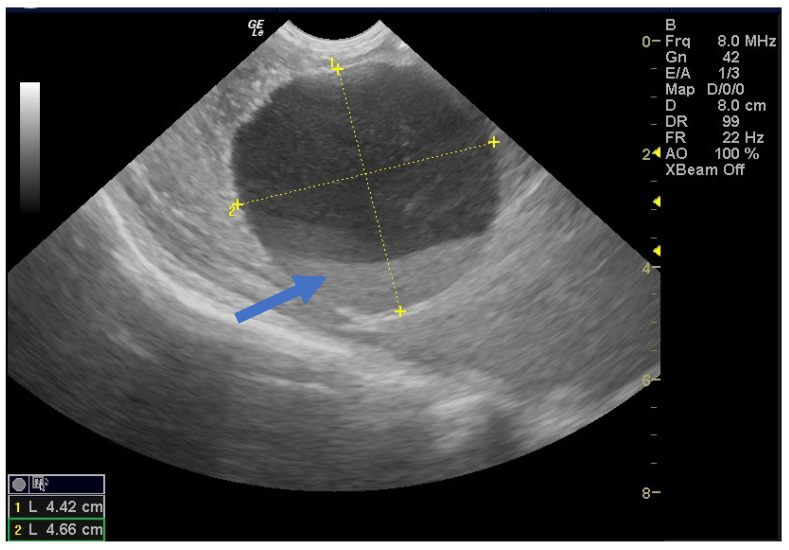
Ultrasound image of a simple cyst showing a blood clot (arrow) that resolved spontaneously after 8 months postpartum.
